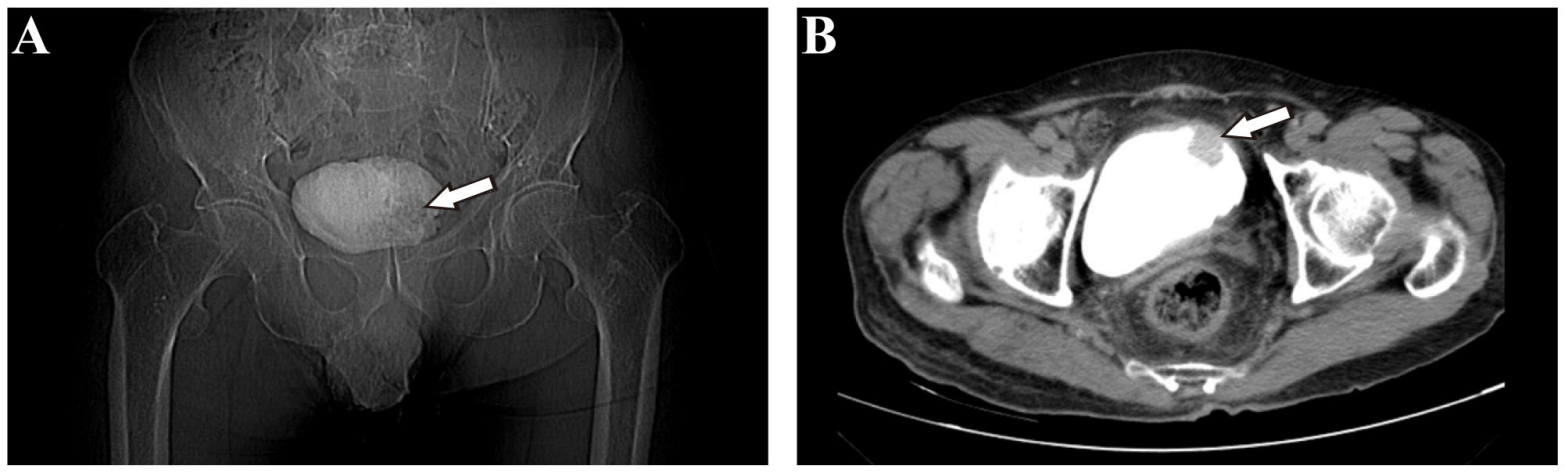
Imaging examination of patients before operation. (A) Coronal view of pelvic X-ray film revealed a shadow on the left bladder wall (white arrow). (B) Axial view of pelvic enhanced computed tomography (CT) revealed a cauliflower-like space-occupying lesion in the left anterior wall of the bladder.
